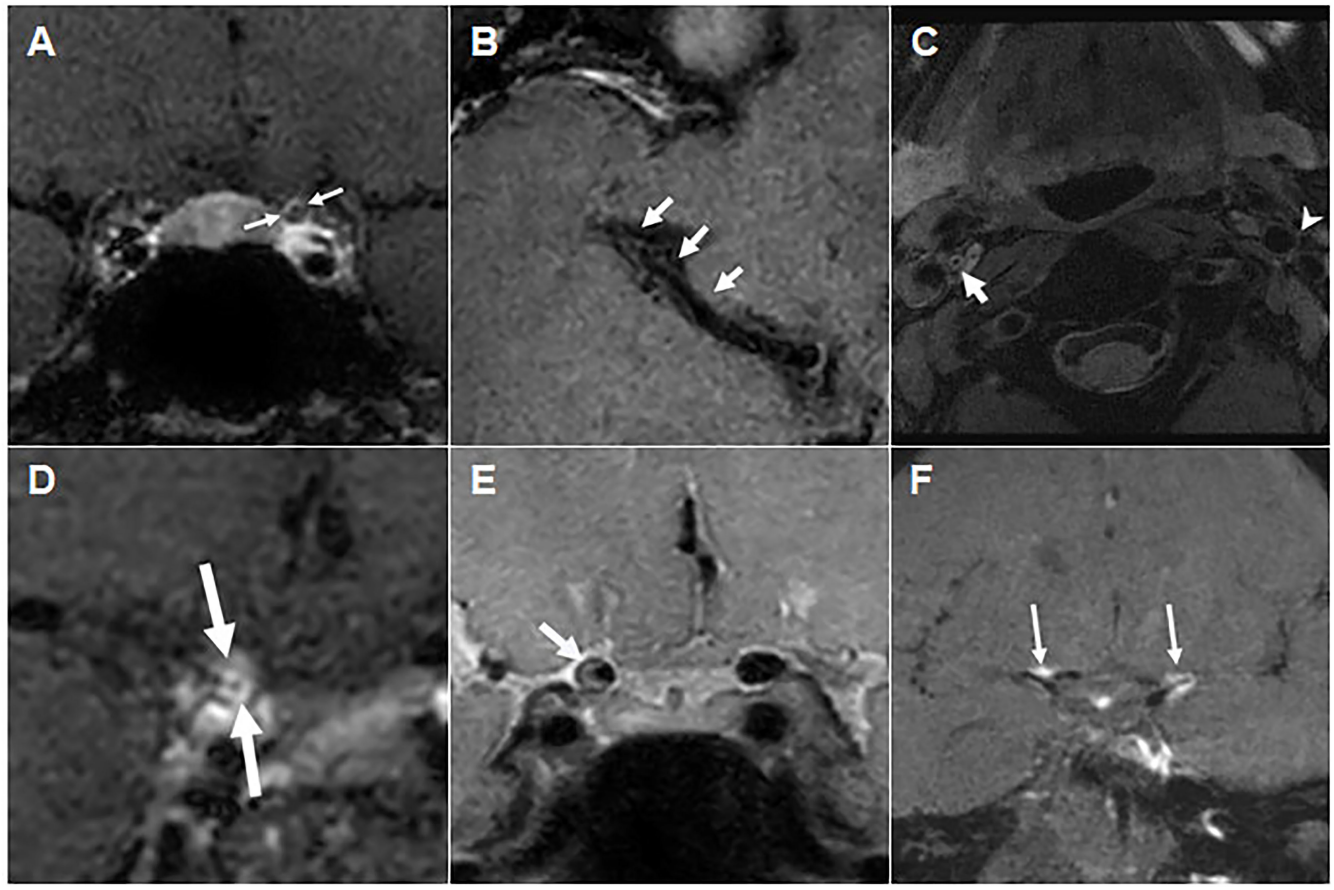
Vascular wall and intraluminal changes on high-resolution vascular wall MRI. (A) Post-contrast T1 weighted image of Patient 1, showing negative remodeling and diffuse concentric wall thickening (arrows) in the left distal ICA, along with mild contrast enhancement. (B) Contrast-enhanced T1 weighted image of Patient 2, showing a diffuse luminal narrowing of the right MCA M1 distal segment without contrast enhancement. (C) Proton density-weighted image of Patient 3, showing concentric intraluminal thickening of the right proximal ICA without definite occlusion and with no overt wall enhancement (post-contrast T1 weighted image not shown). (D) Contrast-enhanced T1 weighted image of Patient 4, showing concentric wall thickening and strong circumferential enhancement of the right ICA supraclinoid segment. (E) Proton density-weighted image of Patient 5, showing eccentric wall thickening with inner eccentric contrast enhancement (post-contrast T1 weighted image not shown) of the right distal ICA. (F) Contrast-enhanced T1 weighted image of Patient 9, showing multifocal vessel wall and subarachnoid nodular enhancement of bilateral ICA bifurcation and left ACA A1 segment (arrows). ACA, anterior cerebral arteries; aPLs, antiphospholipid antibodies; ICA, internal carotid artery; MCA, middle cerebral artery; PV, non-thrombotic proliferative vasculopathy; VW-MRI, vessel wall magnetic resonance imaging.
